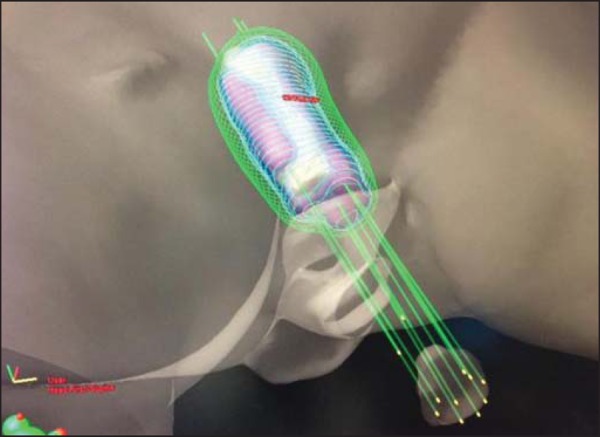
Three-dimensional view based on computed tomography images.
